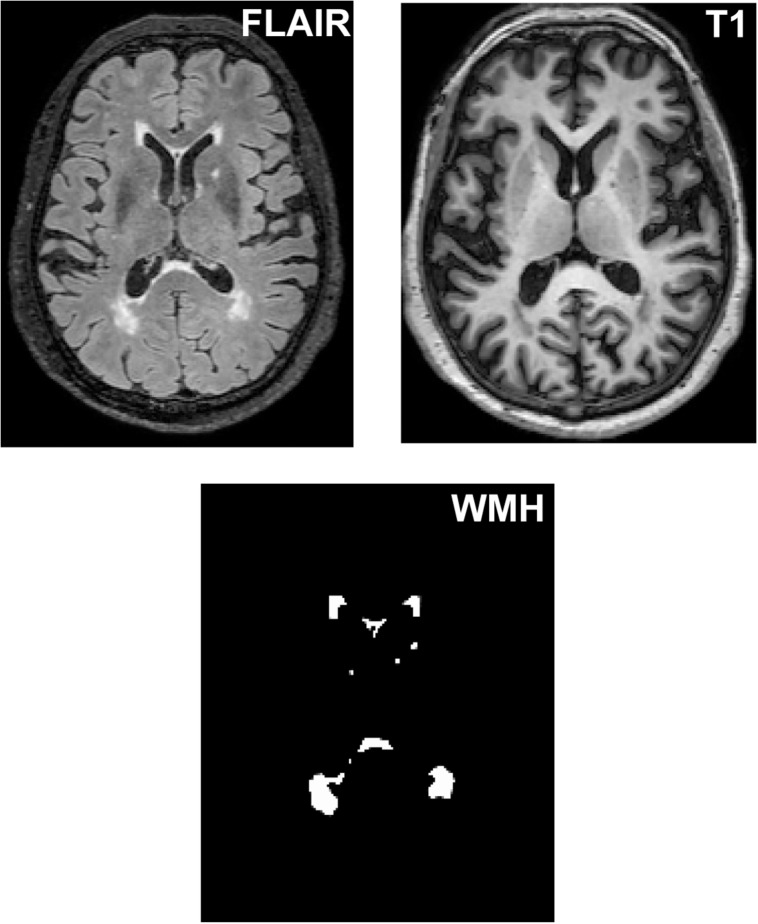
The segmentation of WMH lesions. WMH lesions were segmented and quantified from T_2_ FLAIR images and T_1_ images.
